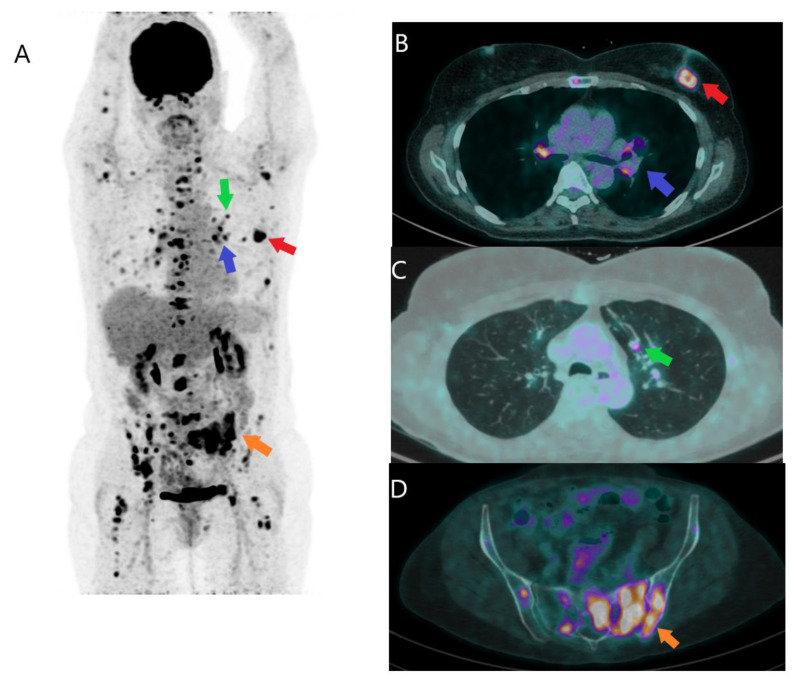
FDG PET/CT in initial staging. A 41-year-old woman with a newly diagnosed triple negative left breast invasive ductal carcinoma of 21 mm, was referred for initial staging with FDG PET/CT. The PET/CT MIP image (A) shows the primary cancer (red arrow), mediastinal and hilar adenopathies (violet arrow), lung metastases (green arrow), and extensive bone involvement (orange arrow). Image (B) (fusion image, trans-axial view) shows the primary lesion of the left breast with necrotic center (red arrow) and the bilateral mediastinal and hilar adenopathies (violet arrow). Image (C) (fusion image, trans-axial view) shows a lung nodule in left upper lobe (green arrow). Image (D) (fusion image, trans-axial view) shows extensive bone involvement of sacrum and ilium (orange arrow).
